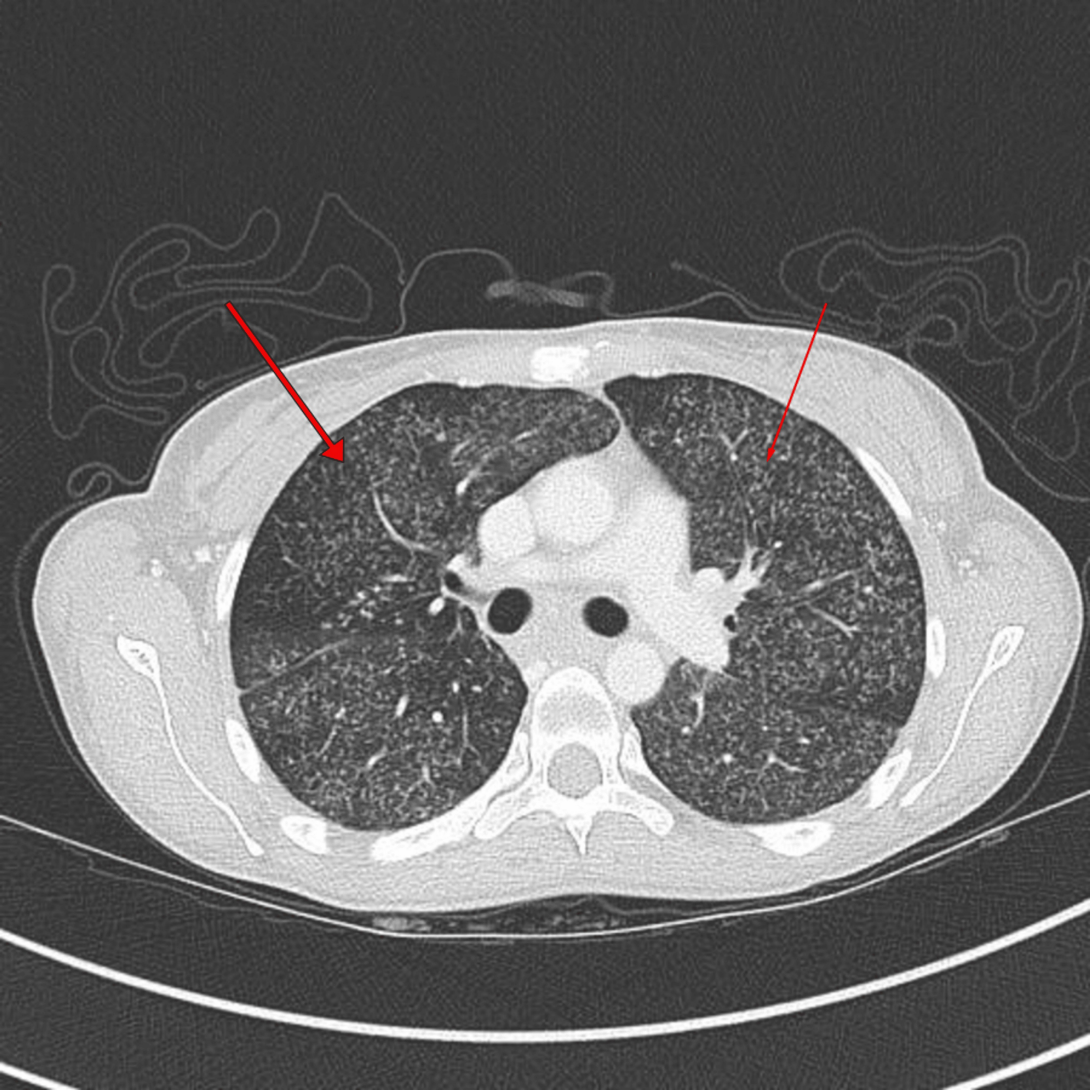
CT thorax showing hematogenously disseminated pulmonary tuberculosis, with arrows pointing to innumerable miliary deposits in both lungs.
